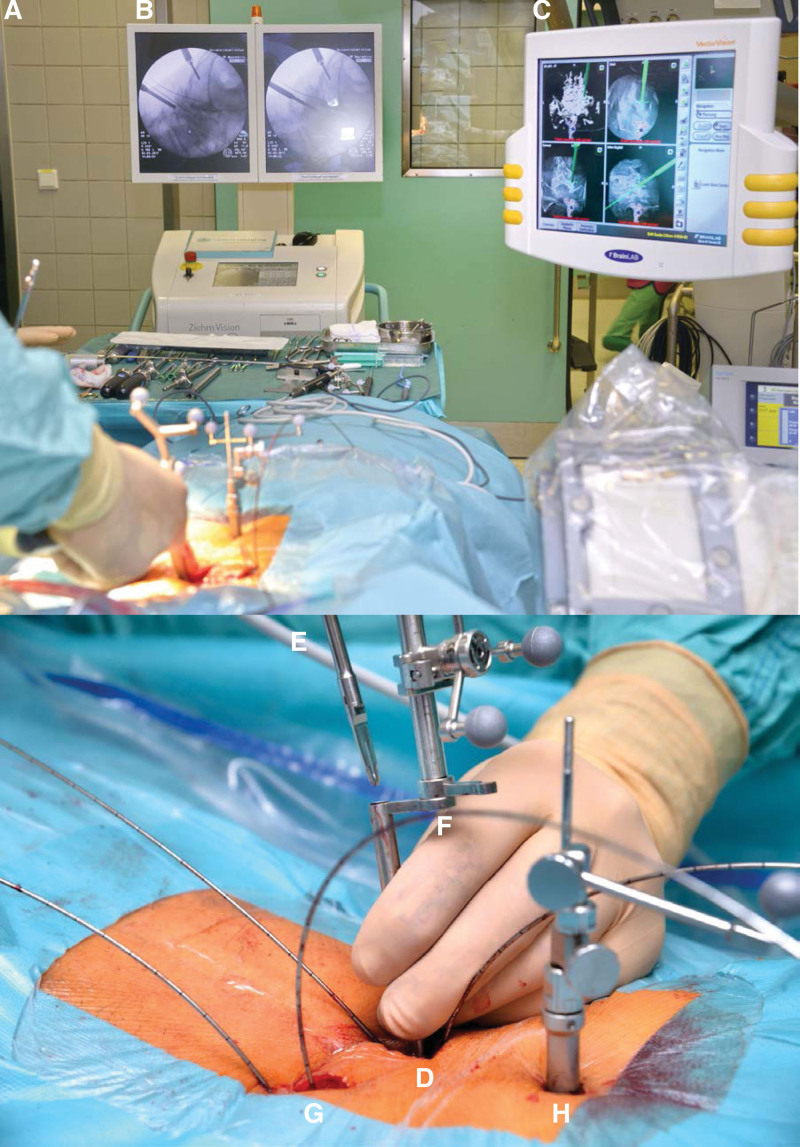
1A–1H. Intraoperative images showing the surgical setting (A) with radiologically guided (B) and navigation-assisted (C) insertion of K-wires. A 3 to 4 cm median incision (D) is sufficient for the laminotomy and ipsilateral and contralateral decompression in crossover technique. In this case, the decompression is done with the help of a navigated ultrasonic knife (E). Through the navigated sleeve (F) fits a high speed drill just as well. Navigated endoscopy armed with ultrasonic knife and high speed drill is another technical feature towards single port endoscopically assisted lumbar fusion because it enables effective endoscopic segmental decompression in an appropriate surgical time. Contralaterally, 2 incisions (G) of approximately 5 to 10 mm are made at a distance of 3 cm from the midline to perform percutaneous pedicle screw placement. Navigation array in situ (H).
